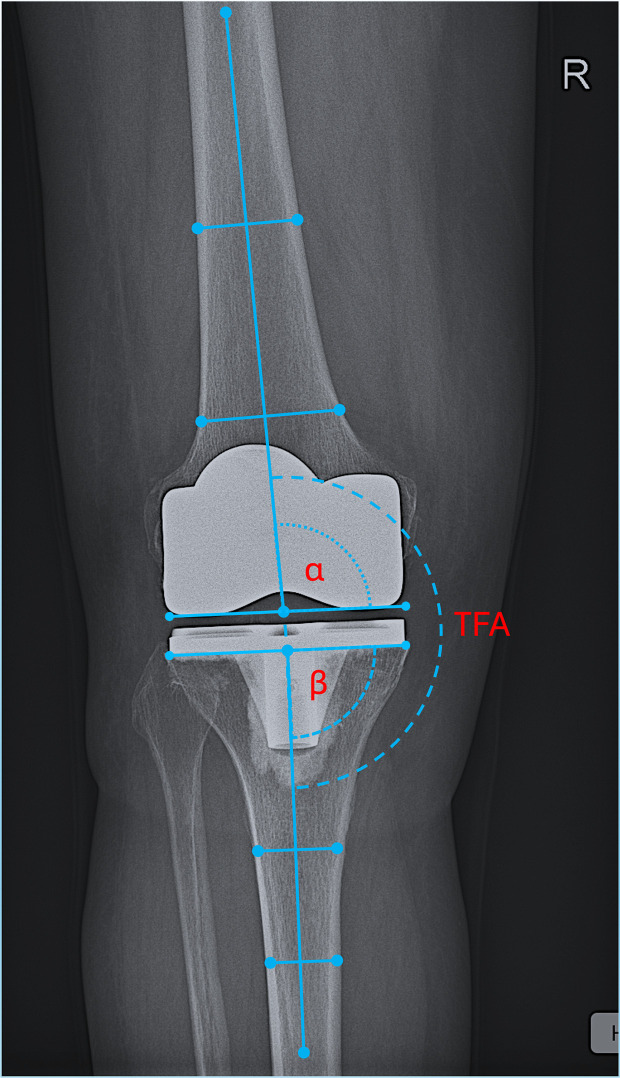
Radiographic measurements of TKA component placement in the coronal plane. Limb coronal alignment is measured by the medial tibio-femoral axis angle (mTFA), defined as the medial angle between the anatomic femoral and tibial axes. A restored neutral mechanical alignment in the coronal plane was considered when the mTFA was 185° ± 3°. Femoral component placement in the coronal plane is measured by the α angle, defined as the angle formed between a line formed by the femoral condyles of the femoral component and the anatomical femoral axis. An α angle of 95° ± 3° represents a correct placement of the femoral component in the coronal plane. Tibial component placement in the coronal plane is measured by the β angle, defined as the angle formed between the tangent line at the base of the tibial component and the anatomical tibial axis. A β angle of 90° ± 3° represents a correct placement of the tibial component in the coronal plane.
